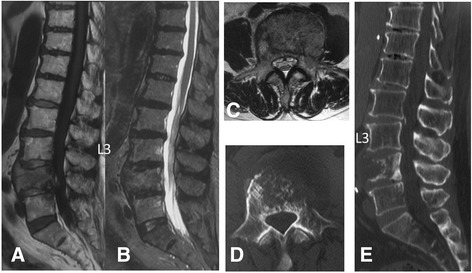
A 64-year-old man with metastasis of liver cancer to the 4th and 5th lumbar vertebrae. The Tokuhashi score was 3. Minimally invasive spine stabilization (MISt) without decompression (L2-S2AI) was applied. The operative time was 182 min, and blood loss was 152 ml. The grade of paralysis improved from D1 before surgery to E after surgery. a Sagittal view on preoperative T1-weighted MRI. b Sagittal view on preoperative STIR MRI. c Axial view at L4 on preoperative T2-weighted MRI. d Axial view at L4 on preoperative plain CT. e Sagittal view on preoperative plain CT
